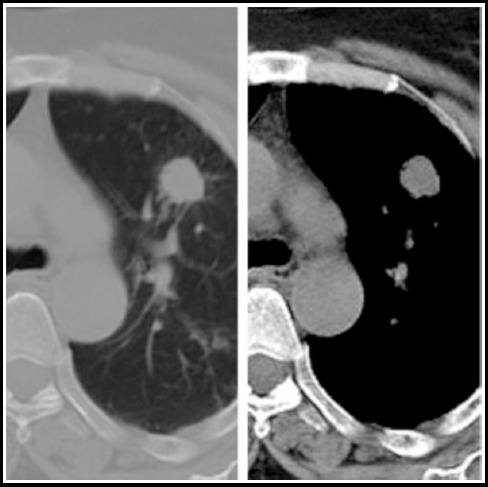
HRCT sections with lung window settings show bilateral cysts with largest thin walled cyst in the right upper lobe.
